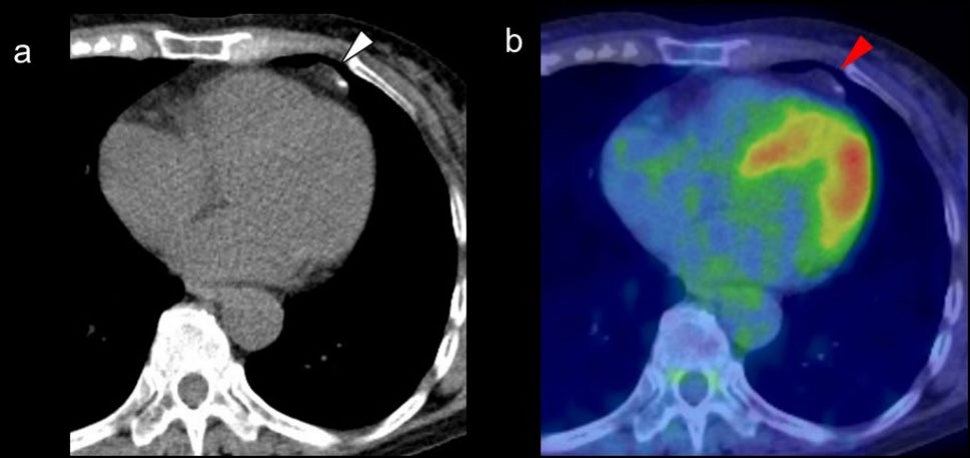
Epipericardial fat necrosis. CT showed small nodular lesion in the epipericardial fat (a white arrowhead), and it demonstrated low FDG uptake (b red arrowhead)
